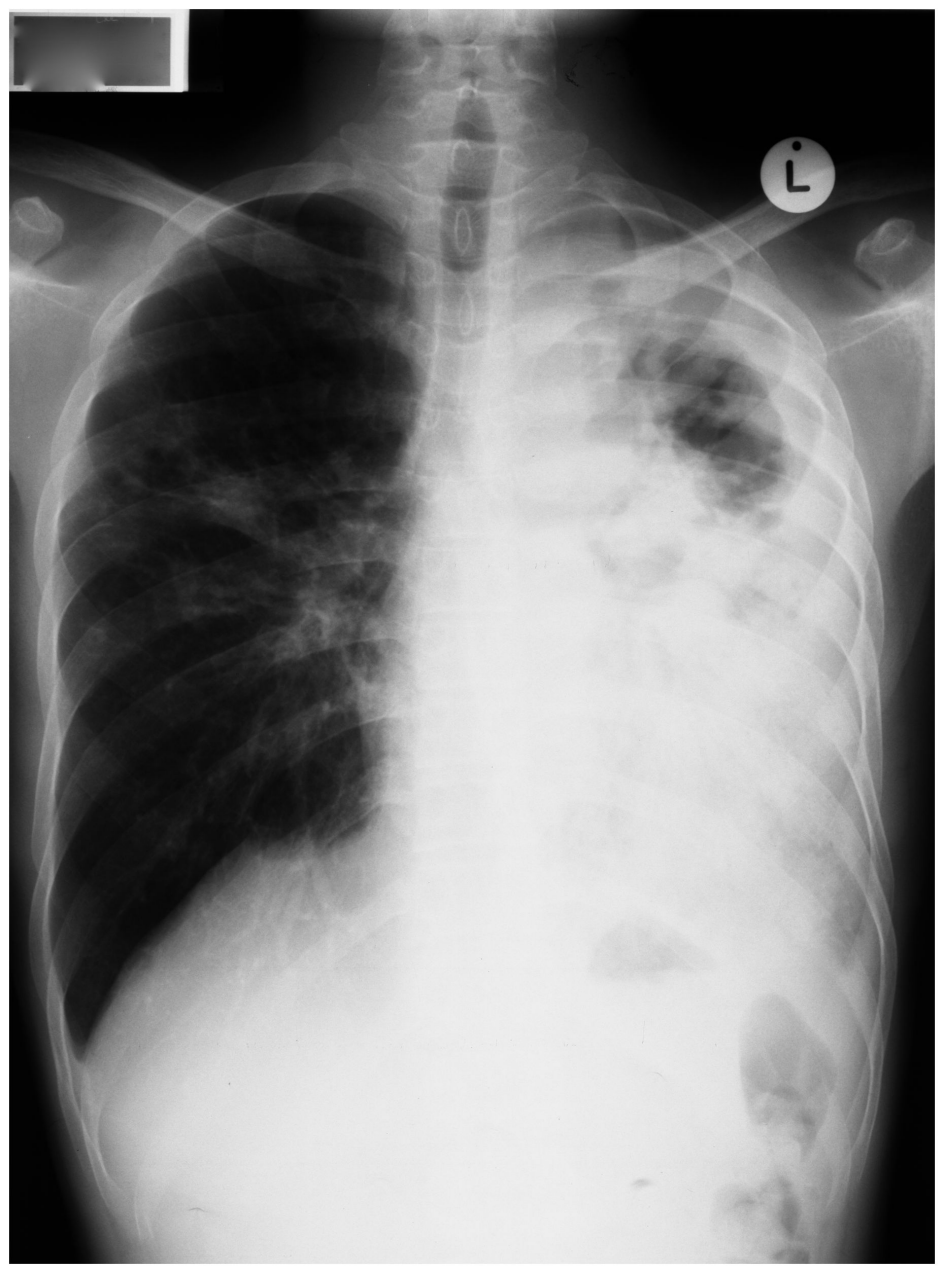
Chest radiograph showing left-sided pleural effusion, consolidation and both hilar and mediastinal lymphadenopathy.
